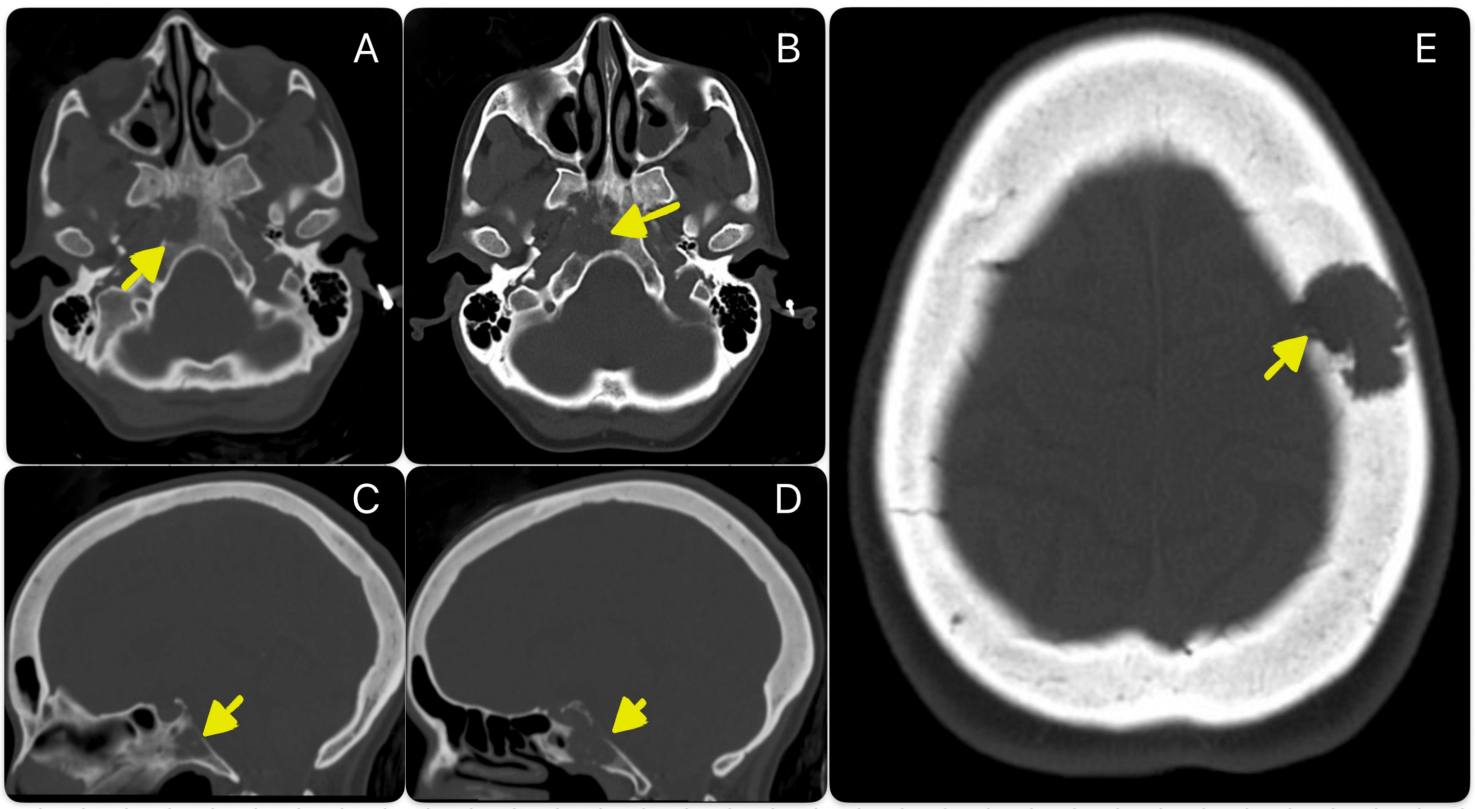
Cranioencephalic computed tomography scan CT scan performed at admission showing a lytic lesion of the clivus (transverse section of cranial CT scan - A, B; sagittal section of cranial CT scan - C, D) and an additional lesion on the left side of the cranial vault (transverse section of cranial CT scan - E).
